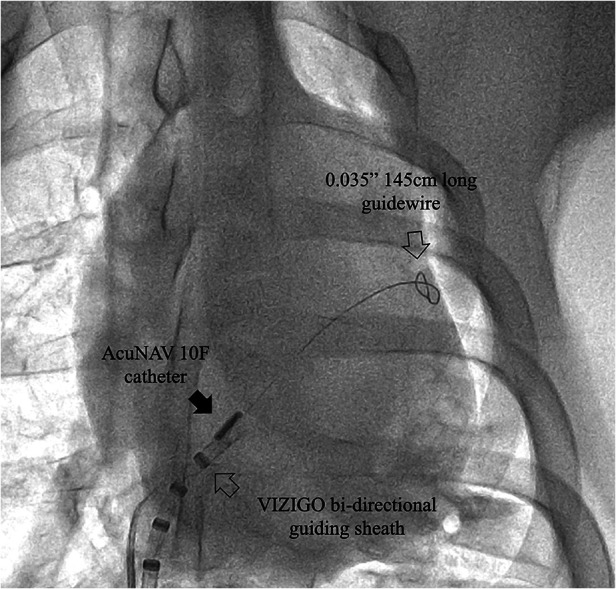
Case 0, x-ray image showing the ICE catheter positioned within the septum area. Note that the tip of the AcuNAV catheter is aligned parallel to the VIZIGO sheath and faces the site where the long guidewire passes through the atrial septum. The sheath and guidewire serve as markers in this process.
